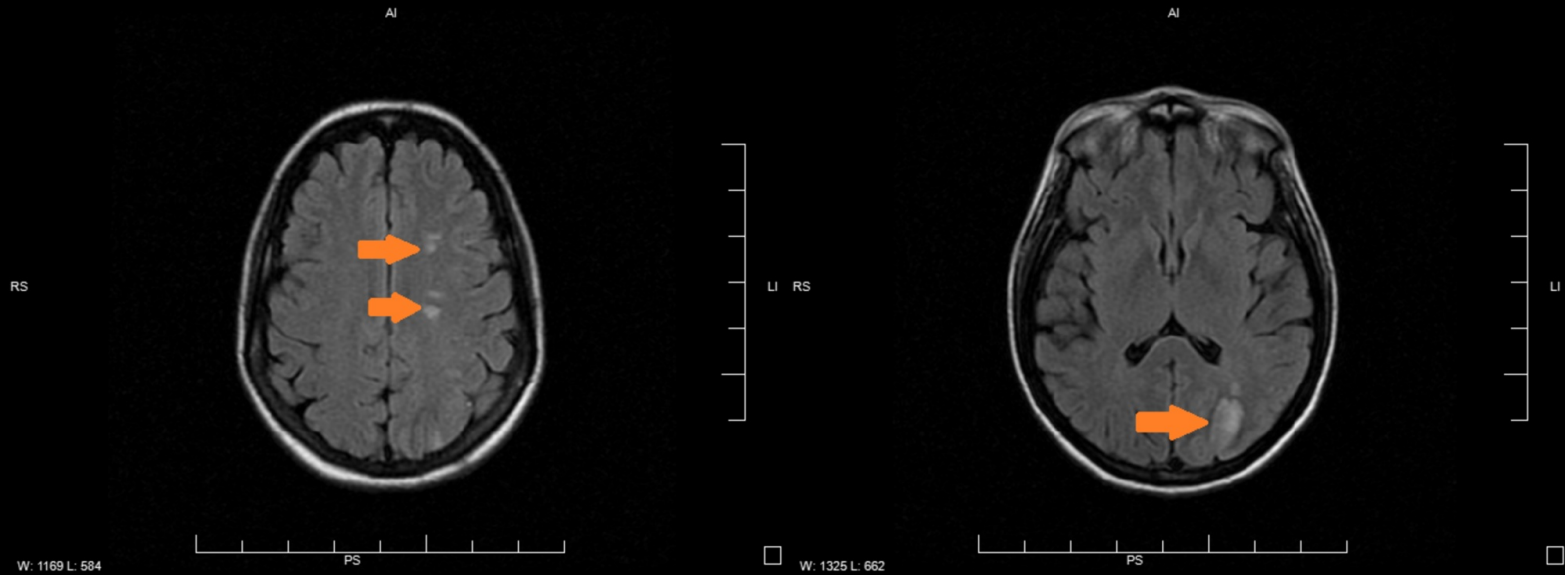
MRI brain: multifocal left hemispheric acute infarcts along the watershed distribution (orange arrows)
